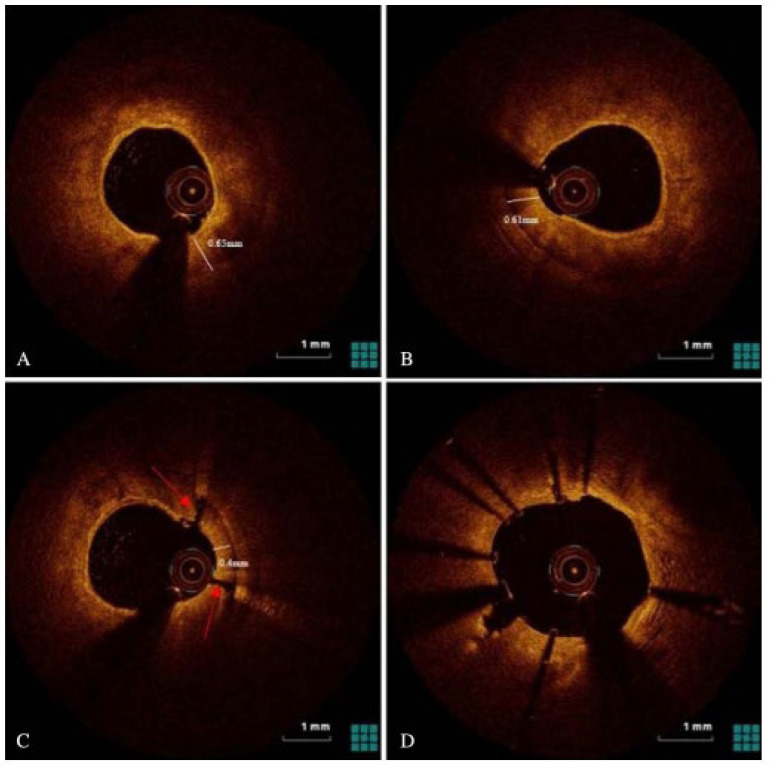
Imagery of optical coherence tomography (OCT)-guided rotational atherectomy (RA). (A) Phase of pre-PCI OCT images depicting heavy plaque calcification with a 360-degree calcium arc and minimal calcium thickness of 0.65 mm. (B) Post-RA step utilizing a 1.5 mm burr with calcium thickness measuring 0.61 mm without any obvious crack formation in plaque structure. (C) Upsizing the burr tip to 1.75 mm, calcium thickness became 0.40 mm with apparent cracks forming (red arrows) in the calcium plaque. (D) After stent implantation with post-dilatation, utilizing OCT imagery detailing successful stent expansion. Source: Teng, W.; Li, Q.; Ma, Y.; Cao, C.; Liu, J.; Zhao, H.; Lu, M.; Hou, C.; Wang, W. Comparison of optical coherence tomography-guided and intravascular ultrasound-guided rotational atherectomy for calcified coronary lesions. *BMC Cardiovasc. Disord.*
